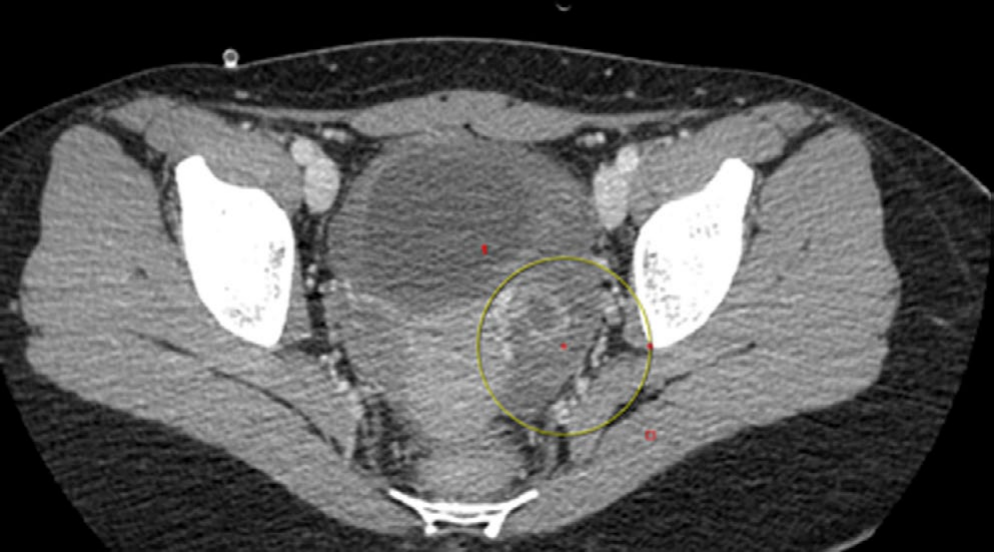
CT image: Presence of cystic mass surrounded by edematous stroma and engorged blood vessels
